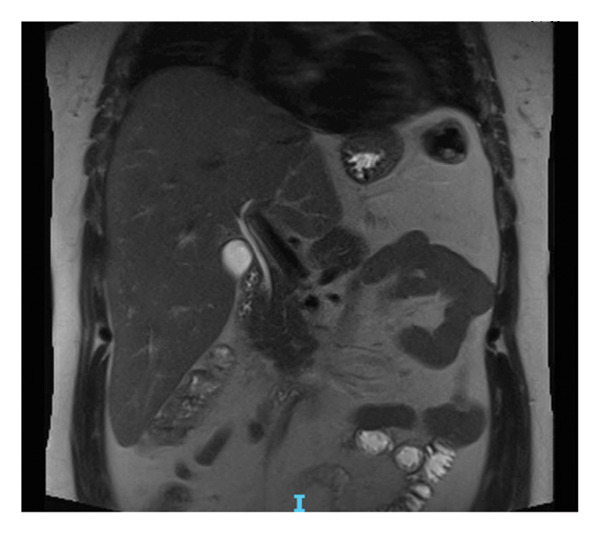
Imaging confirming the absence of recurrence 11 years following resection. Abdominal T2‐weighted MRI, coronal section.
